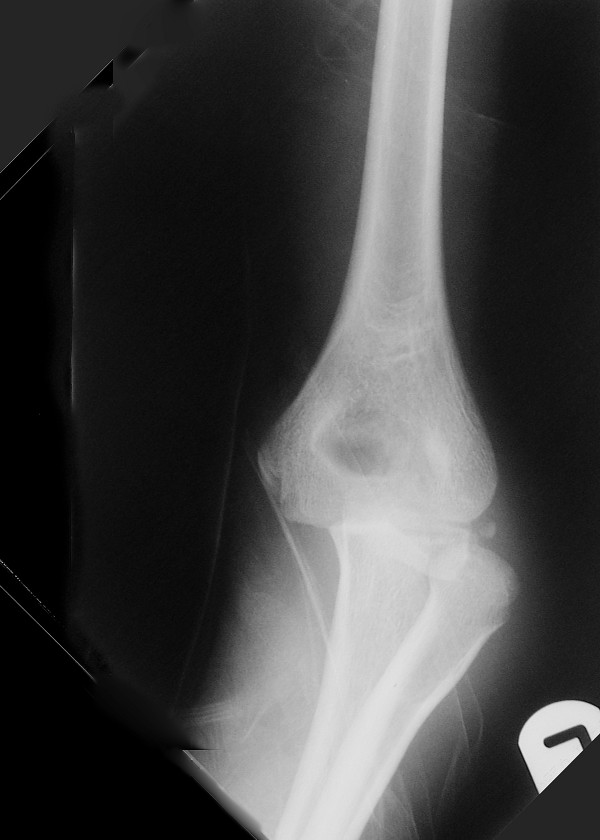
Preoperative anteroposterior radiograph of the elbow revealing fracture lateral condyle in association with posterolateral dislocation of elbow.
